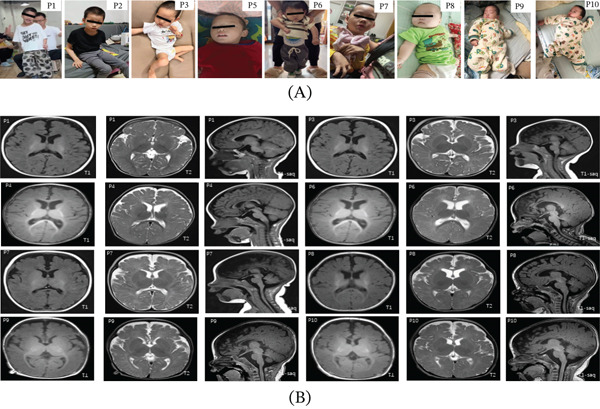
(A) Facial dysmorphisms of representative patients. Patients P1, P2, P3, P5, P6, P7, P8, P9, and P10 exhibit characteristic features including myopathic facies, long face, pale eyebrows, strabismus, large ears, and open mouth. (B) Cranial magnetic resonance imaging (MRI) findings. Images from Patients P1, P3, P4, P6, P7, P8, P9, and P10 are shown (T1‐weighted, T2‐weighted, and T1‐weighted sagittal views). Delayed myelination was observed in all patients. Widening of extracerebral spaces was present in P3, P4, P6, P7, P8, P9, and P10. Thinning of the corpus callosum was noted in P1, P4, P9, and P10.Widened and deepened cerebellar sulci was observed in P9. P1 (20 months) additionally showed delayed myelination, a thin corpus callosum, and intact bilateral ventricular morphology. All images are representative of findings at the time of diagnosis. Scale bars and MRI sequence parameters are provided in the corresponding original radiology reports.
